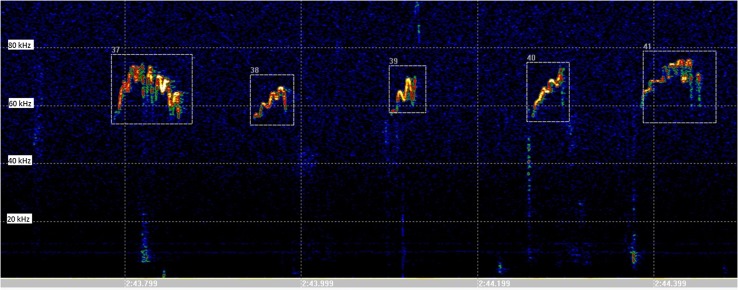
An example of 50-kHz ultrasonic vocalization episodes
